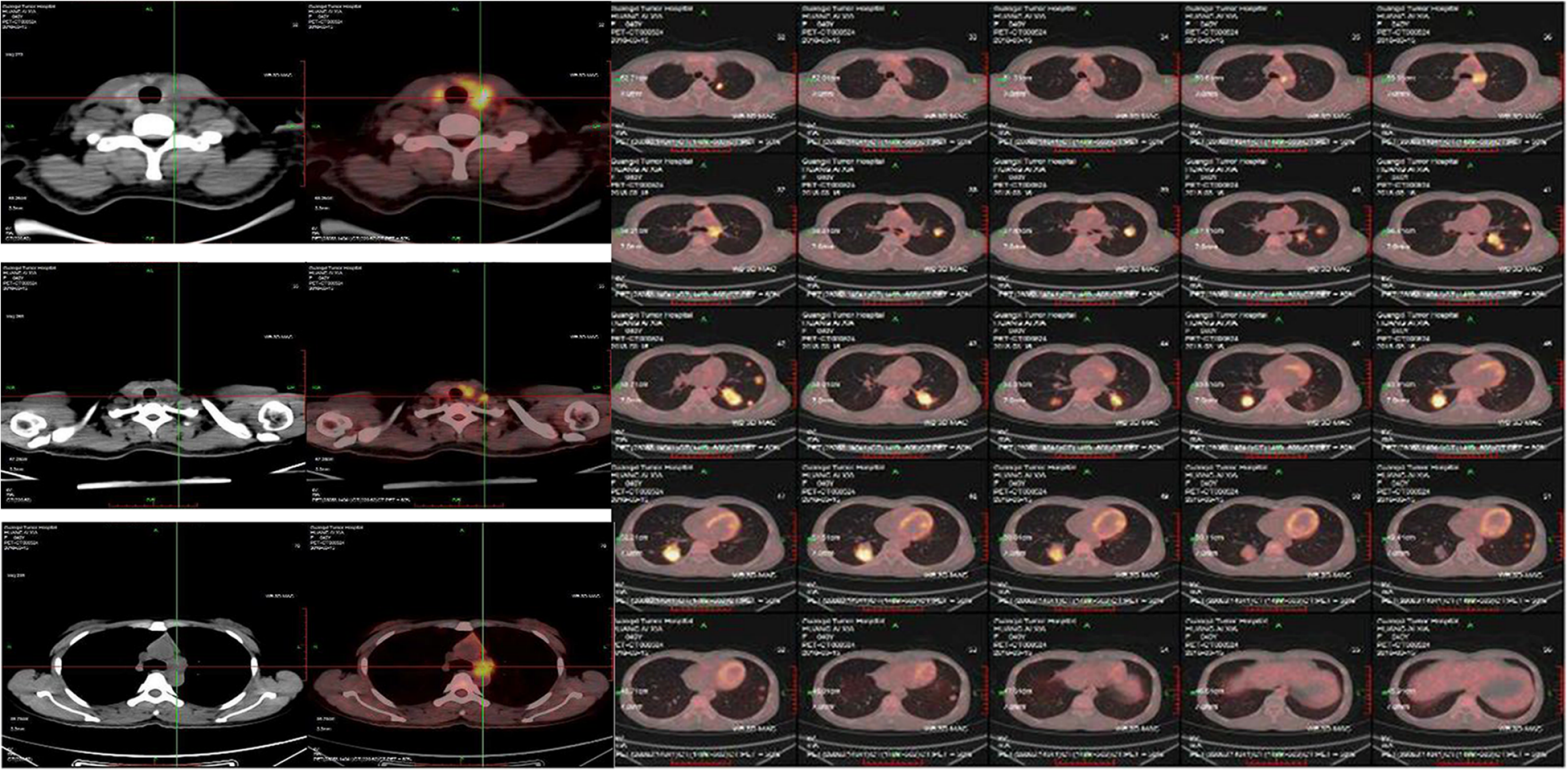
The ^18^F-FDG PET/CT scan images showing increased focal FDG uptake in the multiple thyroid masses, cervical lymph nodes, the fifth region mediastinal lymph nodes and multiple pulmonary nodules
